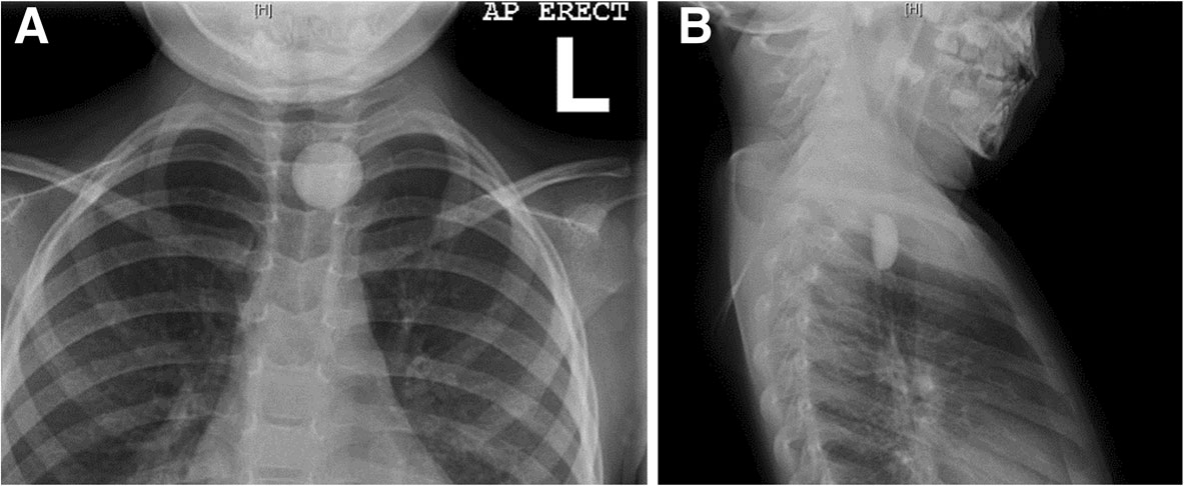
Chest X-Ray of a pediatric upper esophageal foreign body in anterior-posterior (a) and lateral (b) view. Letter “L” indicates the patient’s left side
